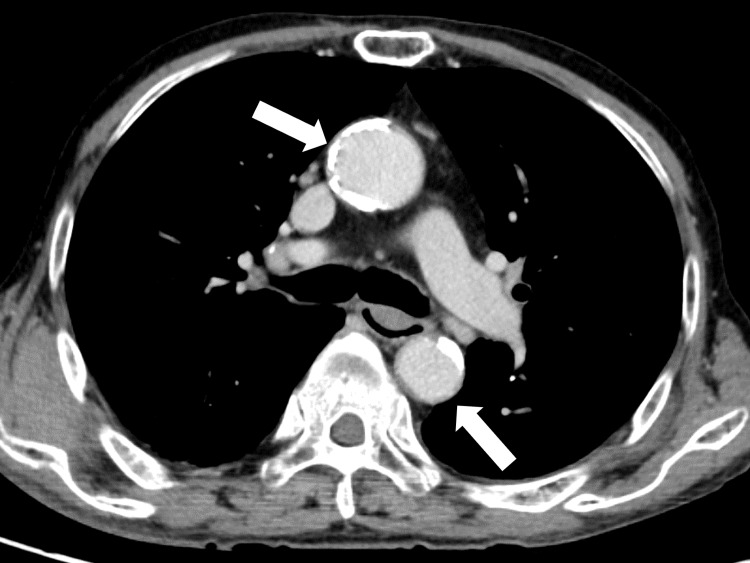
Enhanced chest-to-pelvic computed tomography shows an enhanced and thickened chest aortic wall (white arrows).
